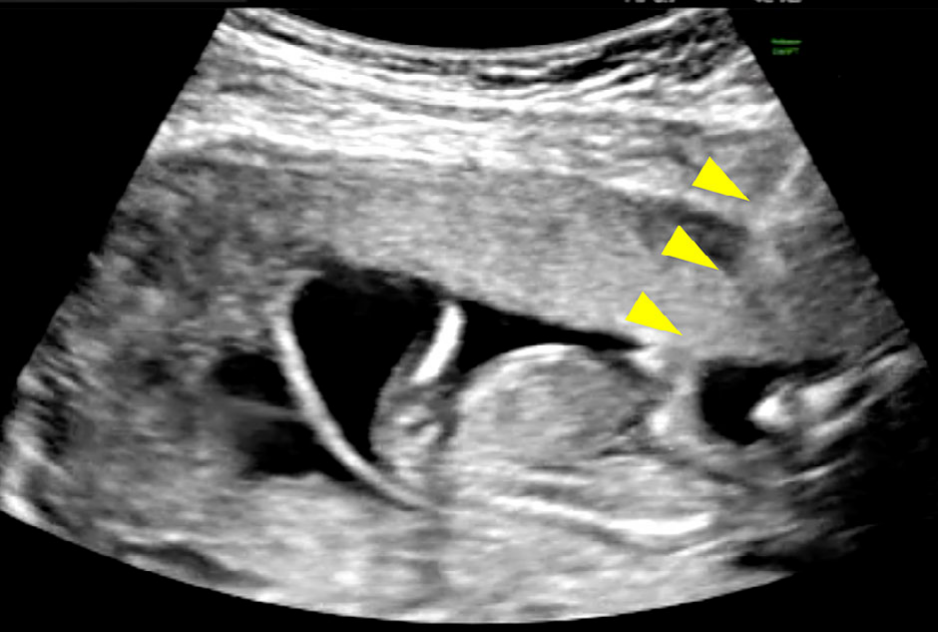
Transabdominal ultrasound‐guided fetal cardiac cavity puncture (arrows indicate puncture needle).
